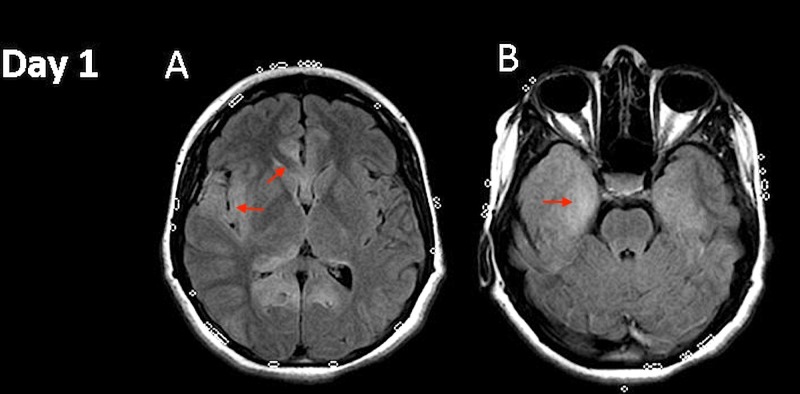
Magnetic resonance imaging (MRI). Hospital day 1 fluid attenuated inversion recovery (FLAIR) changes are noted in the mesial frontal and temporal regions and right insula (A and B).
